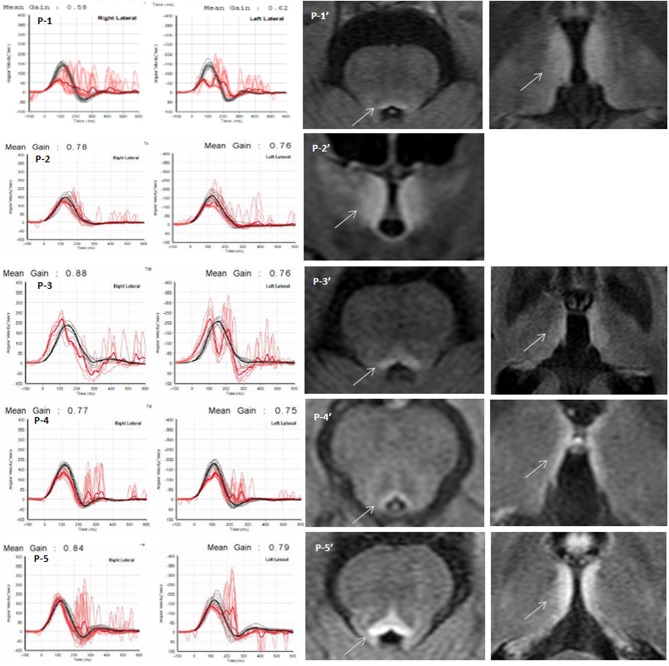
Quantitative video head impulse test (vHIT) and brain MRI. *Left panels*: Within 5 days after symptom onset, eye (in red) and head (in black) velocity traces for right and left head impulse tests of each Wernicke's encephalopathy patient are plotted against time (20 trials per canal) (P1~P5). Vestibulo-ocular reflex (VOR) gain (mean ± standard error) of each patient was significantly reduced, and overt catch-up saccades developed during passive head impulses. Note that eye velocity traces are inverted to allow for better visualization and comparison with head velocity traces. Mean gain values (eye velocity/head velocity) are shown in the upper part of the figure. *Right panels:* Brain magnetic resonance image (P1′~P5′) studies (fluid-attenuated inversion recovery images) show bilateral symmetrical lesions (white arrows) in the medial vestibular nucleus, periaqueductal region, around the hypothalamus, and periventricular regions of the thalamus.
